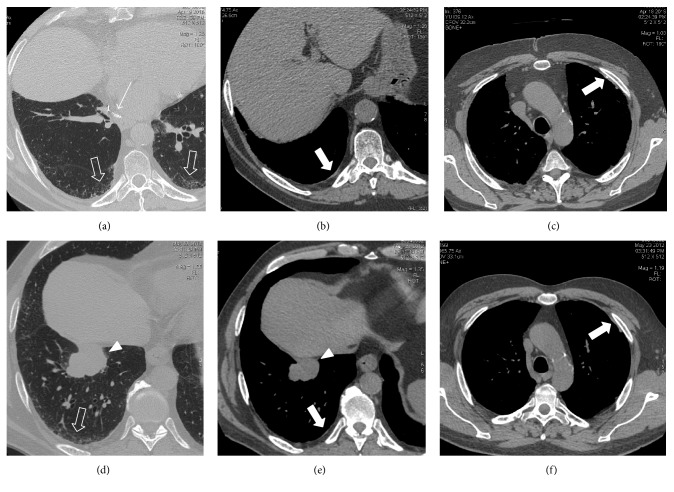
(a–f) Follow-up CT in a 65-year-old man 4 years after lower right lobectomy for lung cancer (arrow in (a)) demonstrates asbestosis (white open arrows in (a)) and bilateral PPs (white solid arrows in (b) and (c)). These CT findings were present since 2012 as showed by the presurgical staging CT (lung cancer, arrowhead in (d) and (e); asbestosis, white open arrows in (d); PPs, white solid arrows in (e) and (f)).
